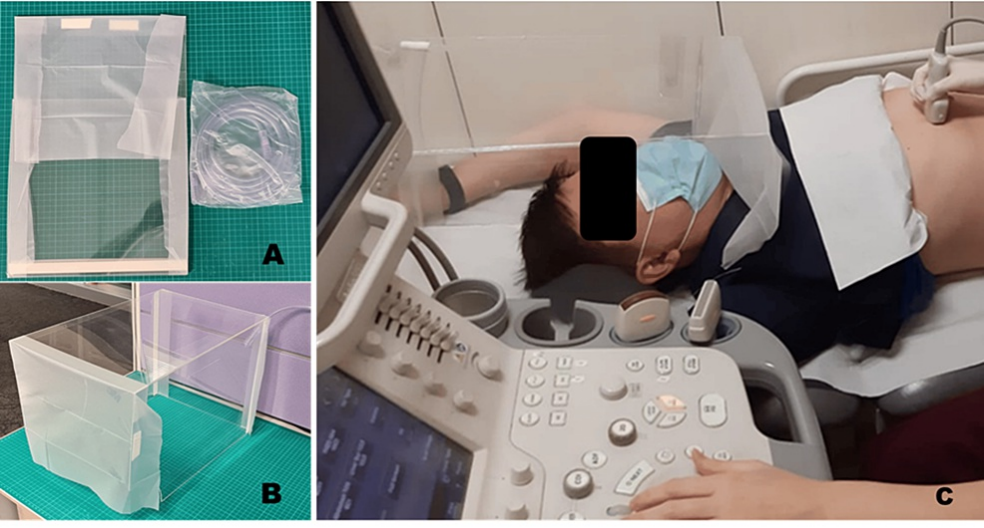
SIR Flat CAP in collapsed flat packed form (A); SIR Flat CAP assembled and ready for use with suction tubing attached via the top right-hand corner (B); Use of SIR Flat CAP in abdominal ultrasound examinations (C)
